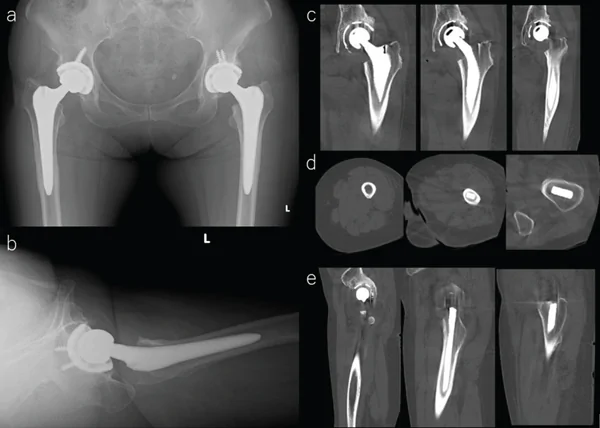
Routine radiographs and computed tomography scans obtained on Day 7 after total hip arthroplasty. No evidence of stem subsidence or periprosthetic fracture was seen on (a) an anteroposterior radiograph, (b) a cross‐table lateral radiograph, or (c) a coronal, (d) axial, or (e) sagittal computed tomography scan.
